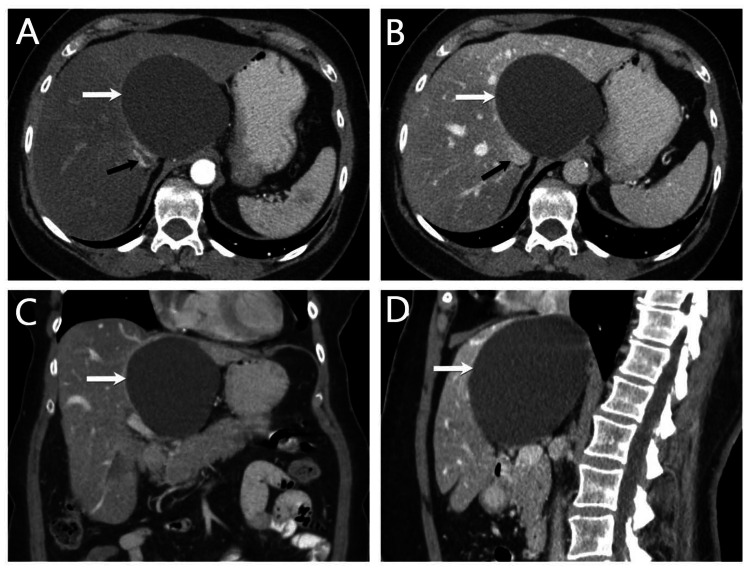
Abdominal CT scan Axial arterial phase (A) axial venous phase, (B) coronal venous, (C) and sagittal venous, and (D) CT images of the abdomen show a large circumscribed hypoattenuating cystic structure (white arrows) within the caudate lobe of the liver measuring up to 10.7 cm, causing a significant mass effect on the surrounding structures, including the IVC (black arrow) and hepatic veins. There is a background of moderate hepatic steatosis. No vascular invasion or lymphadenopathy.
